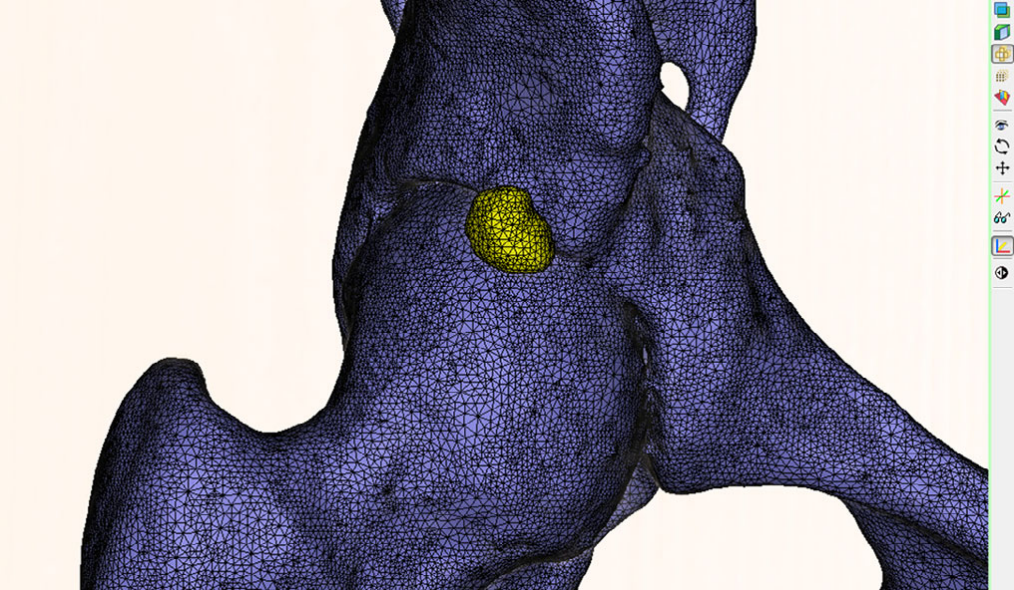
Use of MIMICS software to reconstruct CT scan data and measure labrum calcification volume. The yellow hyperplasia at the edge of the acetabulum was the labrum calcification.
